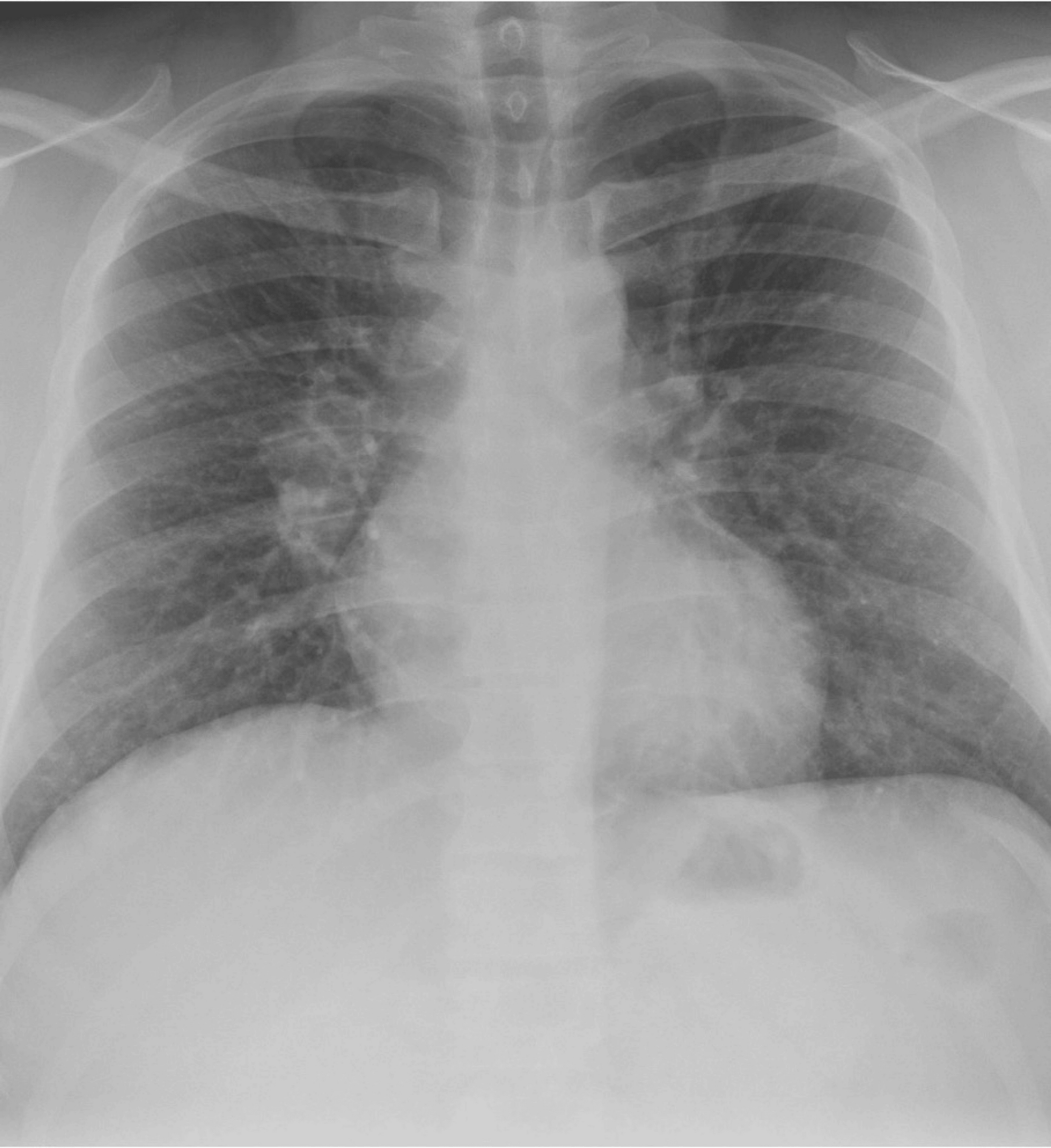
Chest radiograph showing bilateral hilar lymphadenopathy
